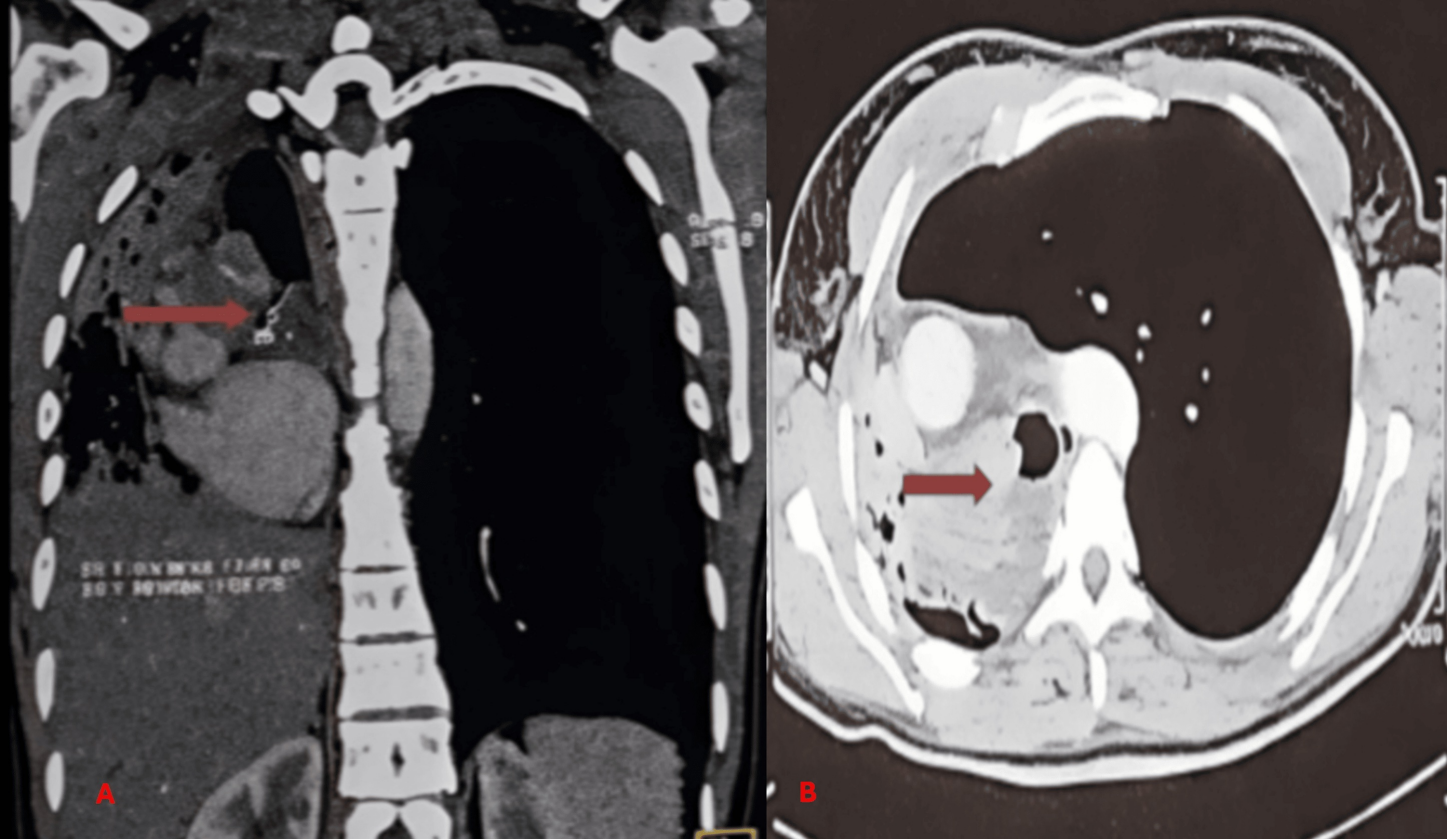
Right hilar lesion on thoracic computed tomography (CT) scan. (A) Coronal thoracic CT image showing a right hilar infiltrative lesion with heterogeneous contrast enhancement (red arrow). (B) Axial CT section confirming the right hilar mass causing bronchial obstruction (red arrow).
